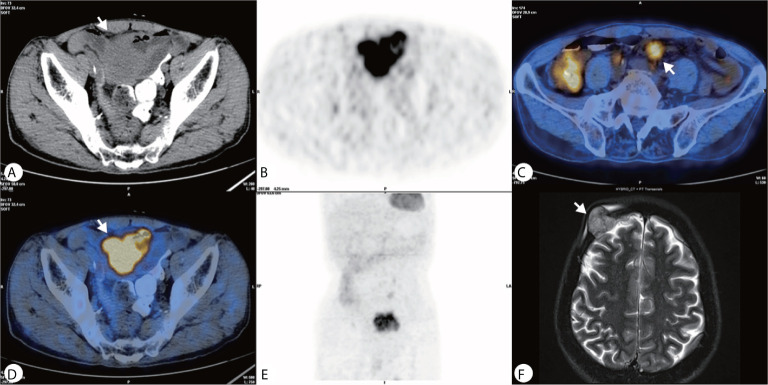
(A–E) PET–CT showed a heterogeneously hypermetabolic pelvic mass, and multiple hypermetabolic nodules were observed in the mesentery; (F) An MRI 2 months after surgery showed an irregular lesion on the right side of the frontal bone, which grew across the skull and invaded the brain tissue.
